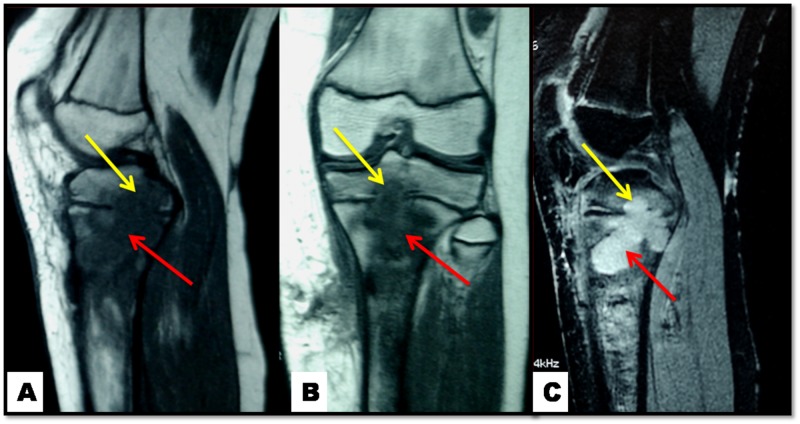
Knee MRI: lytic lesion at metaphyseal (red arrows) and epiphyseal (yellow arrows) regions A: sagittal in T1-weighted sequence; B: coronal in T1-weighted sequence; C: sagittal T2-weighted sequence
